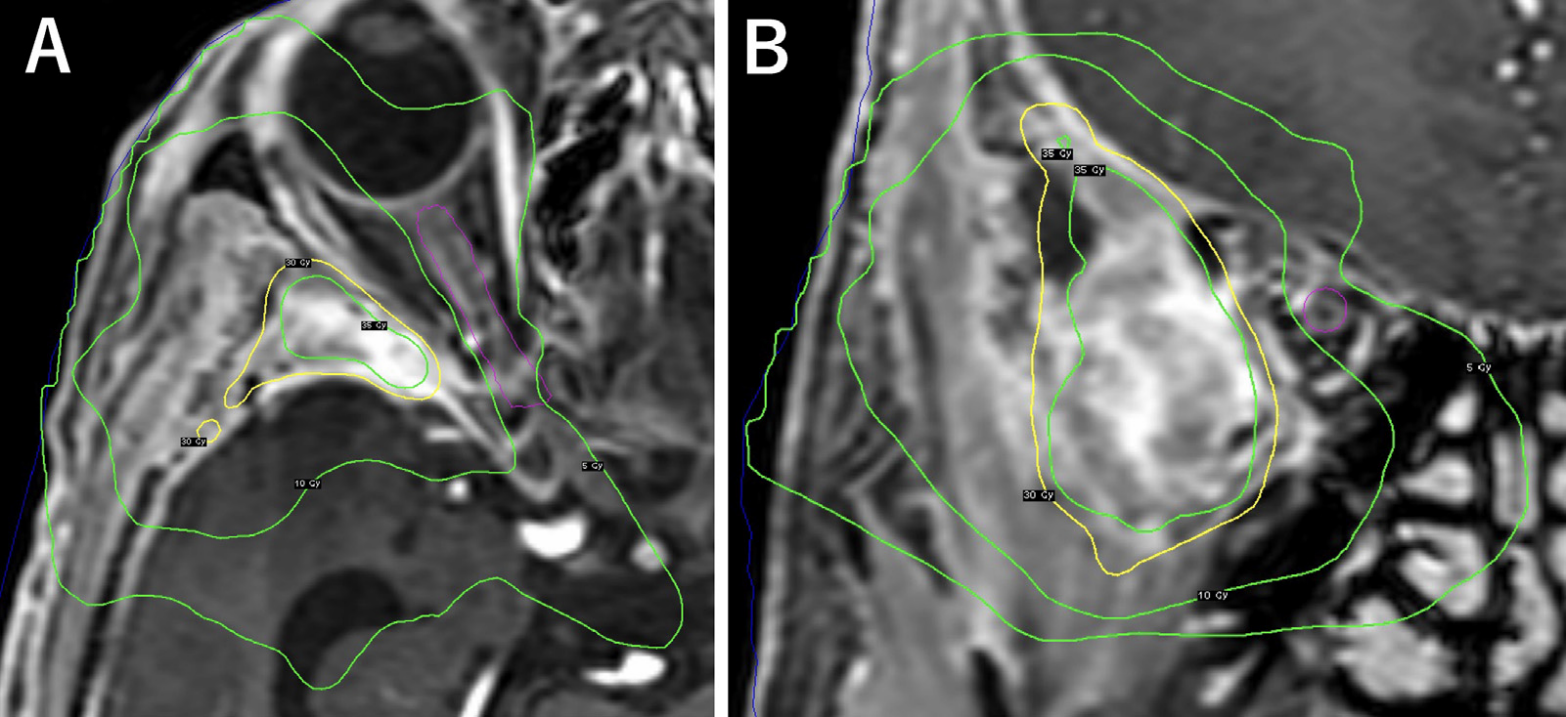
Planed dose distributions on contrast-enhancement T1-weighted axial MR image (A) and coronal MR image (B). Isodose lines indicate from 5 Gy to 40 Gy. The right optic nerve is set as organs-at-risk (pink line).
